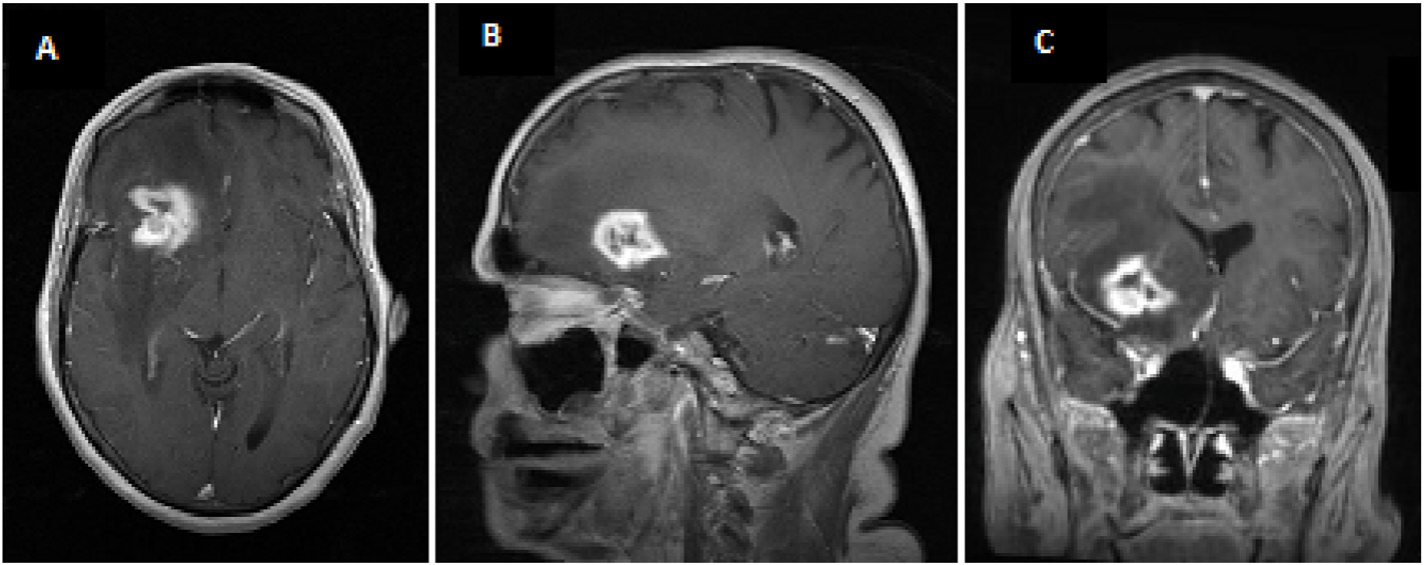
nMeta case - A 55-years old female presents with a recurrent solitary brain metastasis from a mamma carcinoma, six months after resection and local fractionated radiotherapy. Patient has otherwise stable disease without other metastases. She has a Karnofsky Performance Score of 90. Current MRI with gadolinium is shown (A: axial; B: sagittal; C: coronal). Possible treatment choices were: surgical repeated resection, systemic or immunotherapy, best supportive care.
